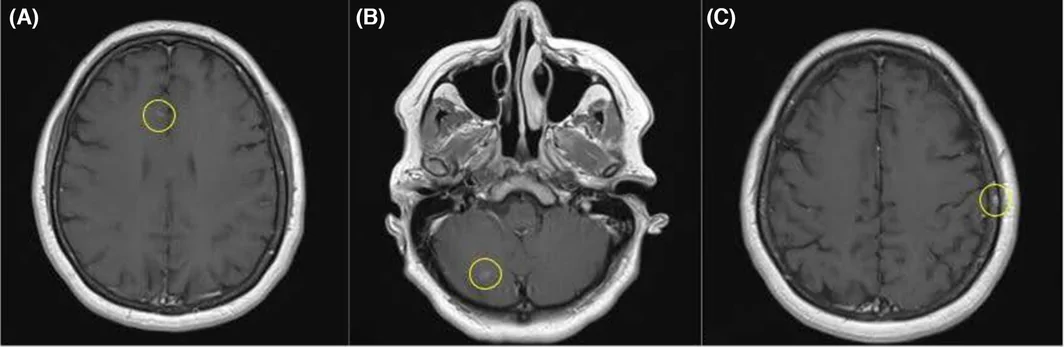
Post‐contrast axial T1 MRI of the brain with yellow circles indicating (A) 0.3 cm focus of enhancement in the paramedian right frontal lobe; (B) 0.4 cm focus of enhancement in the right cerebellar hemisphere; and (C) 1.3 cm left parietal calvarial enhancing and diffusion restricting lesion. None of the lesions are associated with additional signal on GRE/SWI or T2. [Color figure can be viewed at wileyonlinelibrary.com]
